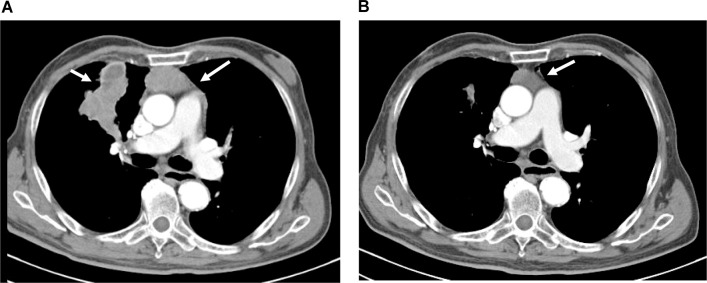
Exemplary CT scans. CT scans of patient number 01–003 were obtained at the beginning of the study (A) and after 12 weeks of treatment with octreotide LAR plus prednisone (B). White arrows indicate tumor lesions.
